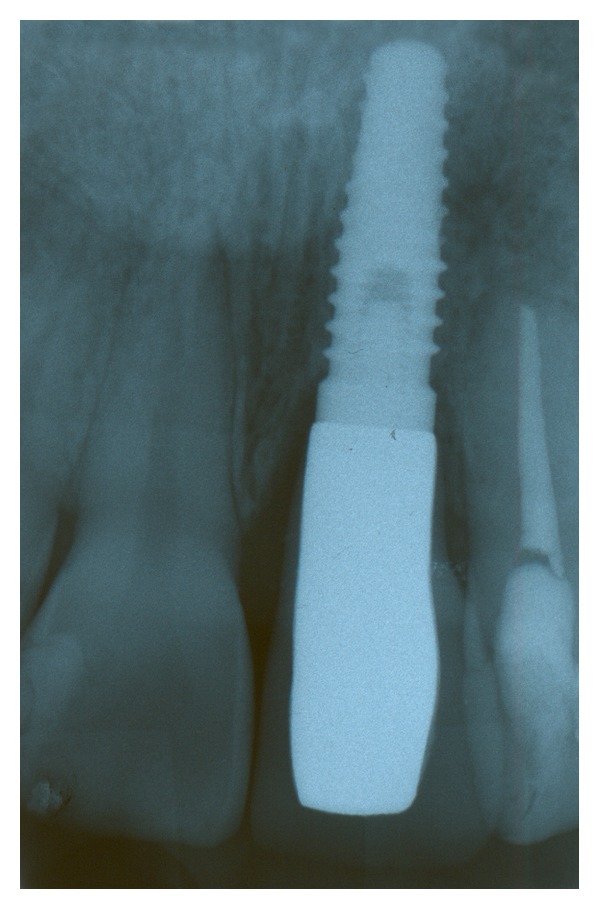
Final radiograph obtained at 6 months after implant placement.
